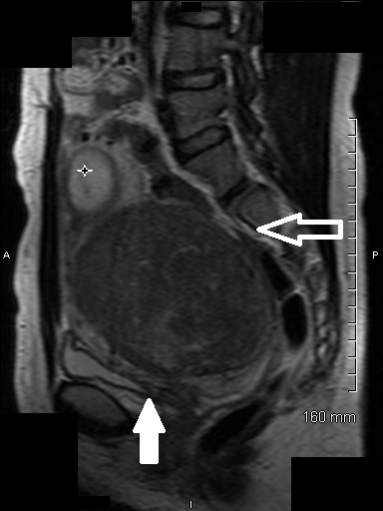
Preoperative magnetic resonance imaging. Magnetic resonance image of her pelvis without contrast shows a gravid uterus with pregnancy in the fundus (star), compression of the colon (empty arrow) and compression of the urethra (filled arrow) that were causing our patient's symptoms.
